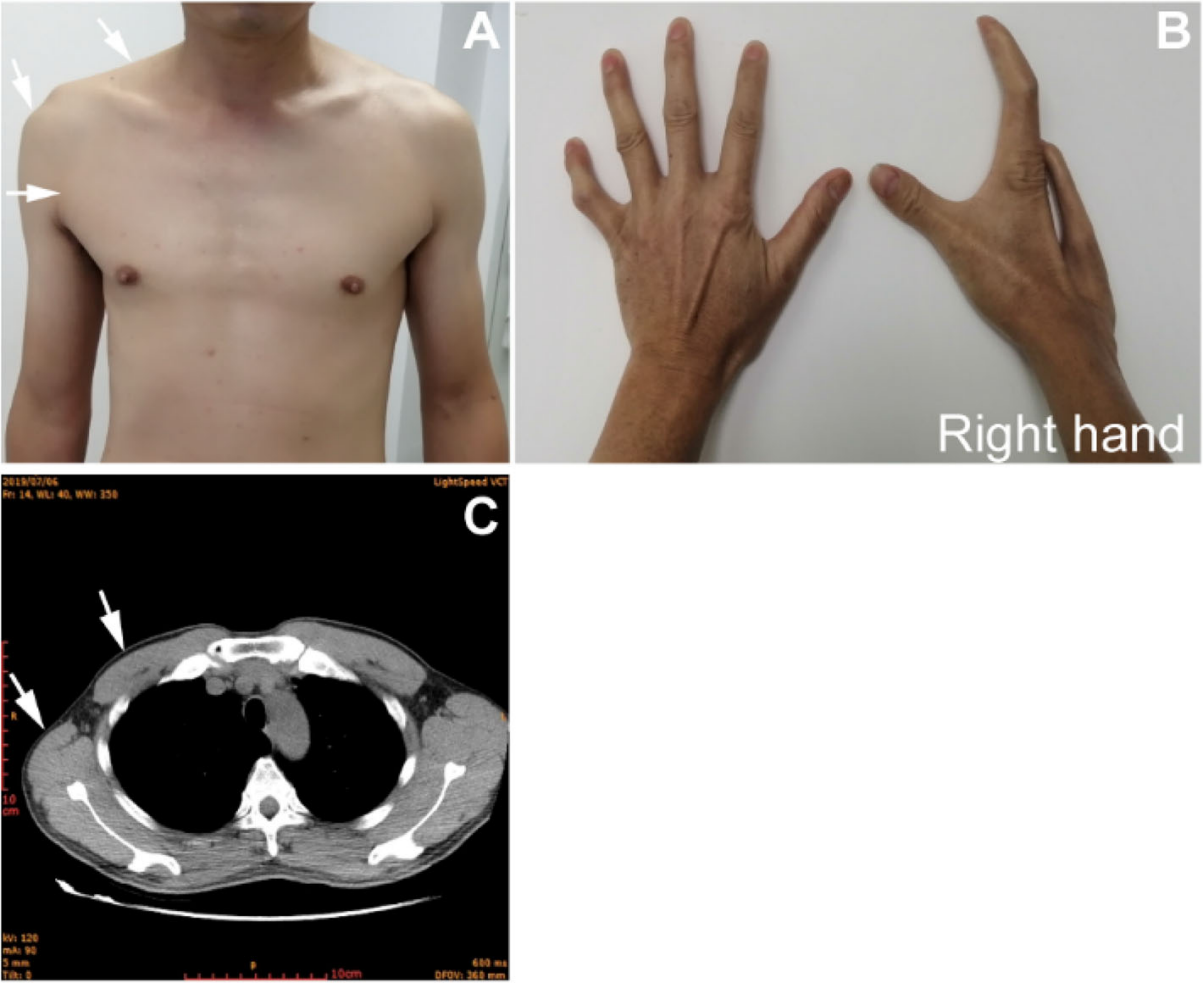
Dysplasia of the chest wall, trapezius, and hand. a White arrows show hypoplasia of the right pectoralis major, trapezius, and deltoid; b deformity of the right hand; c computed tomography demonstrates an incomplete defect of the right thoracodorsal muscle (white arrows)
